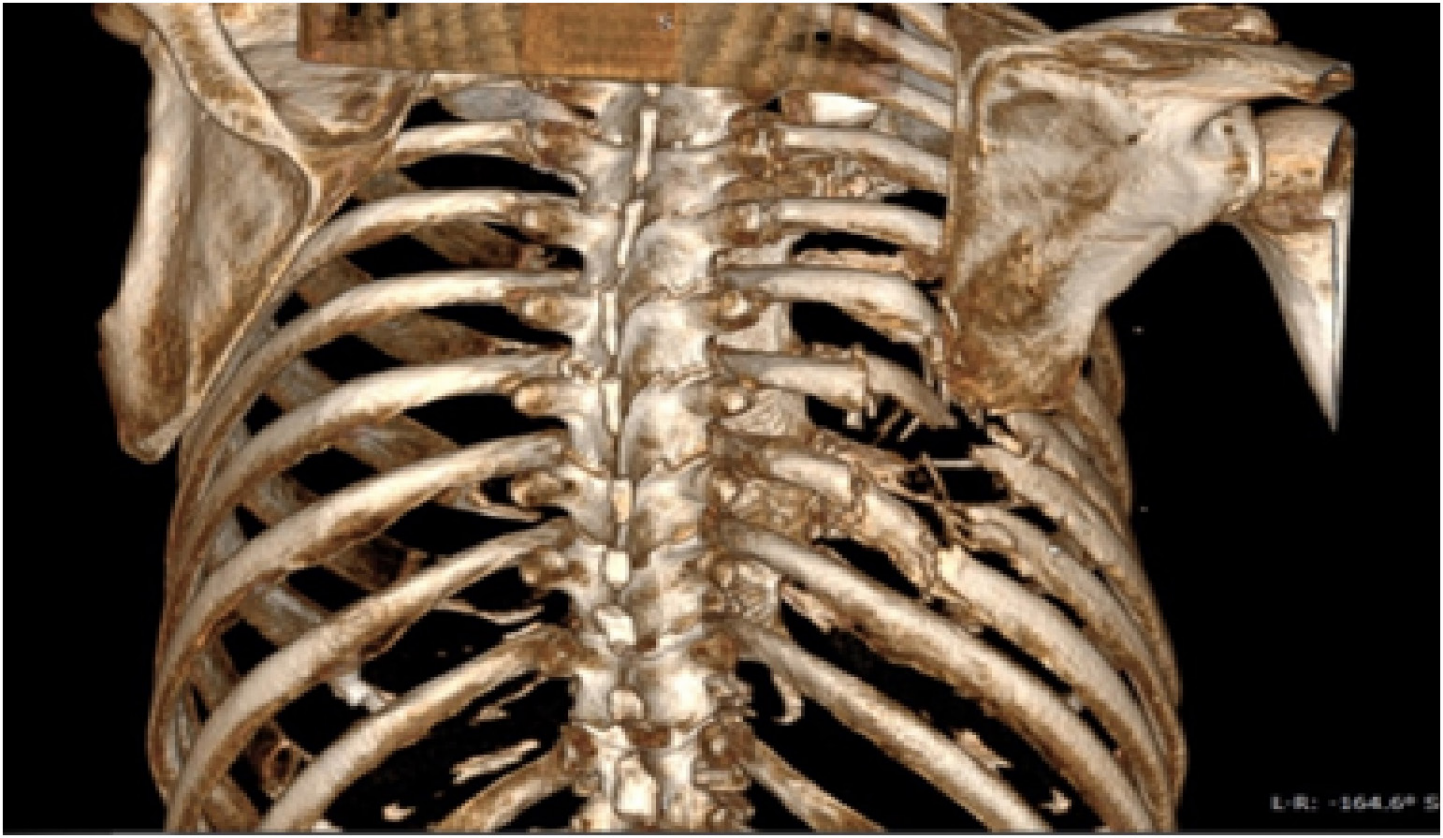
3D CT examination showing the extension of rib fractures.
